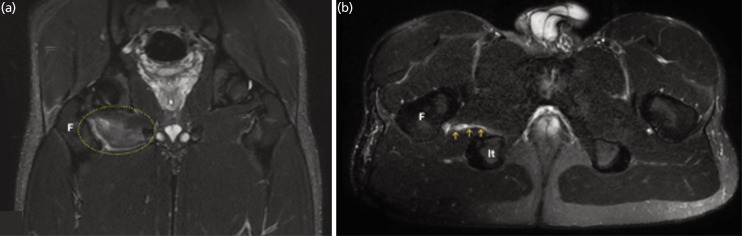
MRI images of moderate indirect right OE tear (STIR and T2 fat sat images). After 2 days from the event, in panel A (coronal, STIR) and B (axial, T2 fat), a moderate lesion of OE muscle can be observed (yellow circle). (a) In particular, a hyper-intense area can be appreciated into the muscular belly. (b) At the site of lesion, a peri-fascial fluid collection (hyper-intense area, yellow arrows), attributable to a sero-hematoma, is also present. (F: femur; It: ischial tuberosity).
